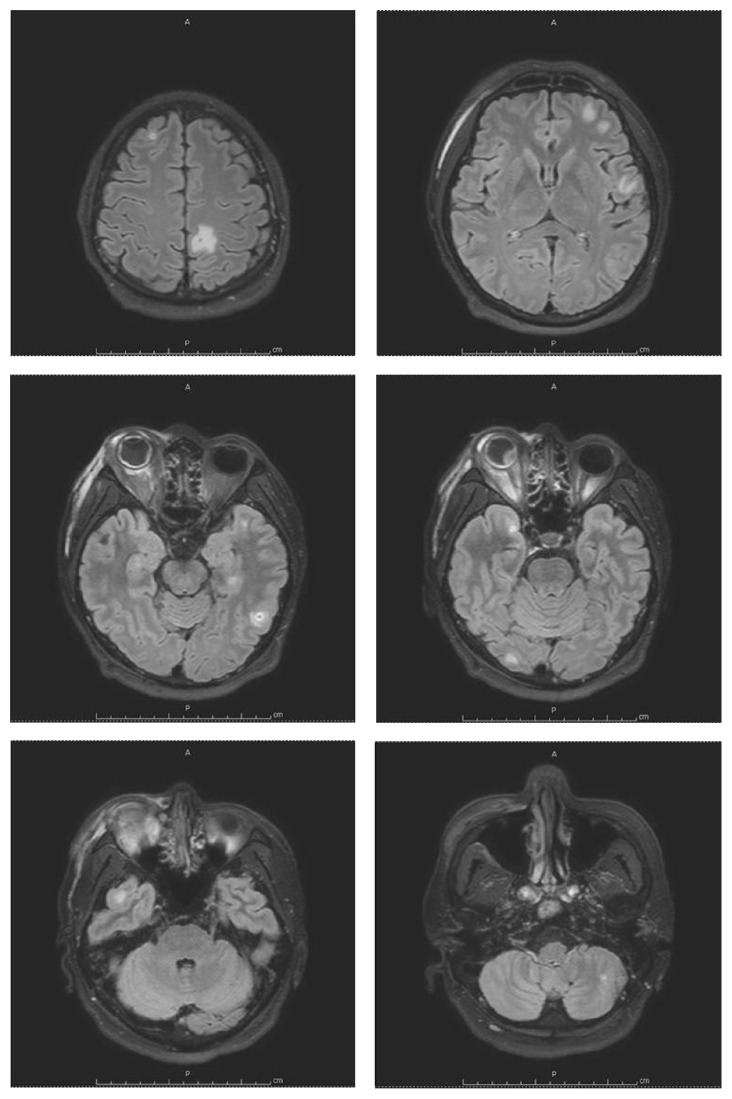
Brain MRI of the patient showing small ring-enhancing hypointense T1/hyperintense T2 lesion at inferomedial aspect in the right orbital globe, enhancement along retina and internal wall of the right orbital globe with suspected hyaloids detachment of the right eye, right exophthalmos, intra- and extraconal fat and periorbital soft tissue enhancement, perioptic neuritis of the right optic nerve without evidence of optic neuropathy, and multiple small round-shape enhancing lesions at gray-white junction of bilateral cerebrum and cerebellum. No leptomeningeal enhancement was detected.
